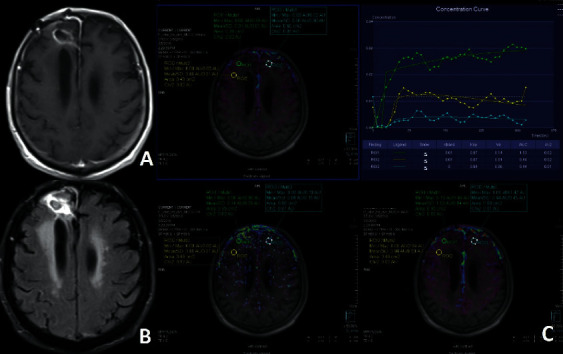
Postcontrast axial T1 (a) and FLAIR images (b) show PT at the ERC in a patient operated for grade 4 glial mass. Ktrans, Ve, IAUC maps, and tissue contrast-time curve of DCE-MRP are seen (c). ROIs are placed at the ERC, NNA, and CLNA(c). Enhancing areas have higher permeability with increased Ve and IAUC but similar Ktrans values compared to the other areas.
